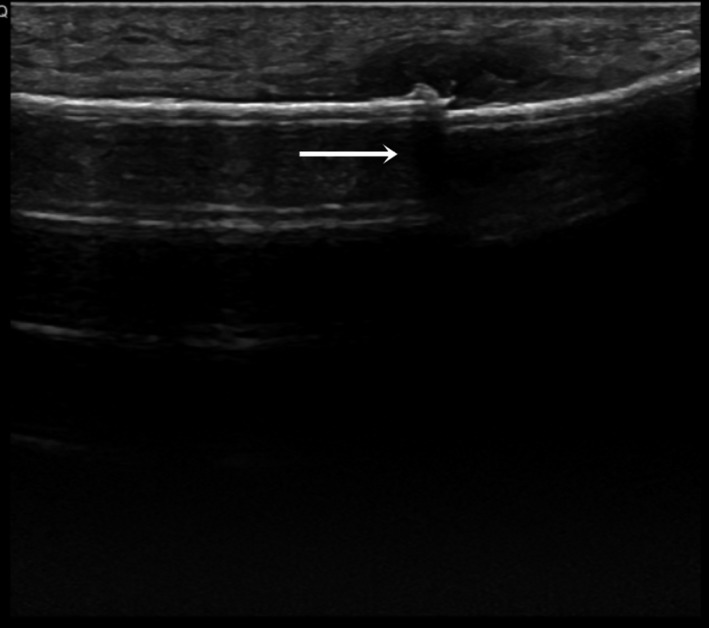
Right leg ultrasound demonstrating fibular cortical discontinuity and soft tissue edema (arrow).
